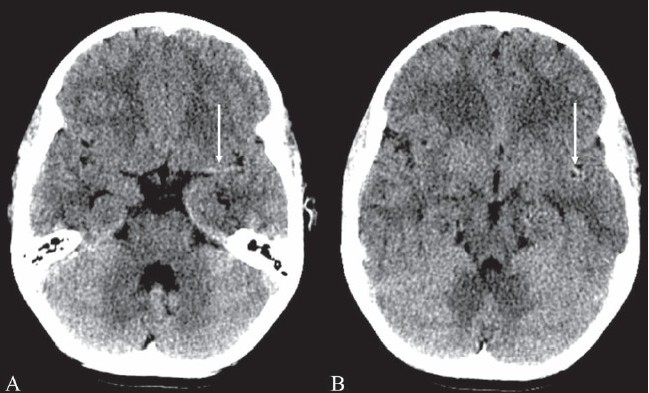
Hyperdense MCA sign. Noncontrast axial CT scans (A, B) of the brain in a patient with sudden-onset right hemiparesis show hyperattenuation of the left middle cerebral artery (arrows). This is suggestive of occlusion of the artery. This is one of the signs of acute stroke but does not necessarily represent infarction
